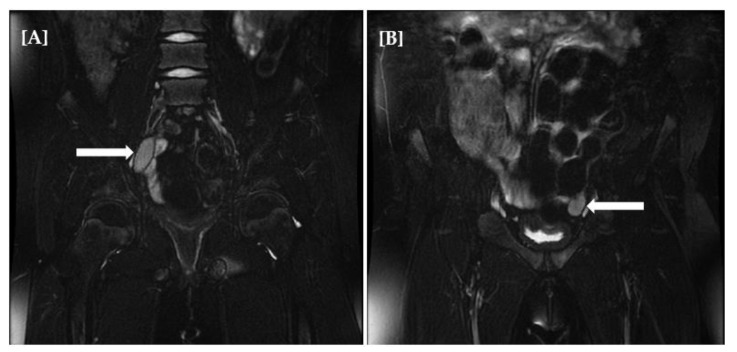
Magnetic resonance imaging (MRI) of abdomen and pelvis minor showing presence of gonads (right (A), left (B)) with the absence of the structures derived from the Müllerian ducts.
